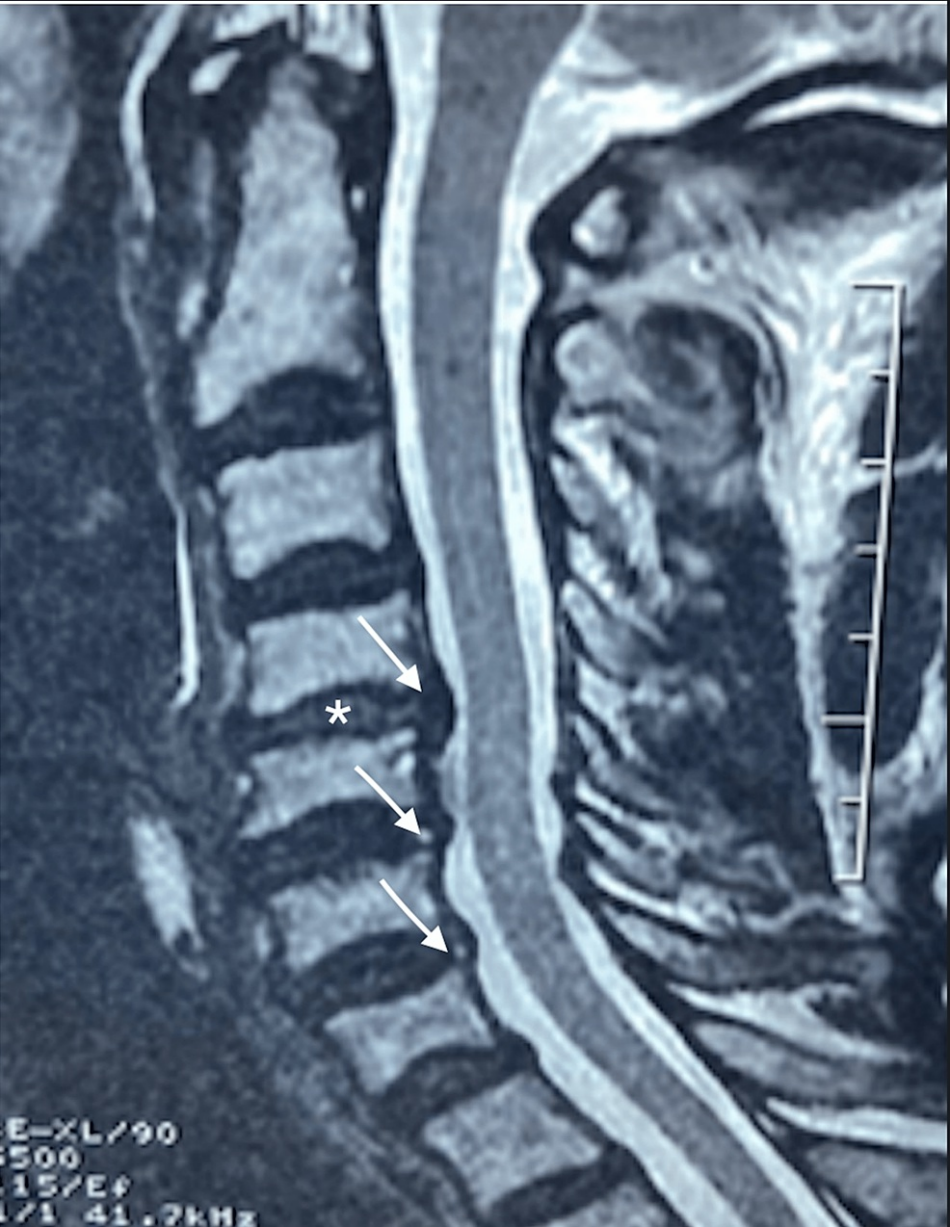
Sagittal T2-weighted magnetic resonance image Sagittal T2-weighted magnetic resonance image revealing loss of the normal cervical lordosis, with endplate changes and osteophytic lipping at the C4-5 and C5-6 levels, loss of disc height at the C4-5 level (asterisk), and posterior osteophyte disc complexes at the C4-5, C5-6, and C6-7 levels (oblique arrows), with no central spinal canal stenosis or cord contact.
